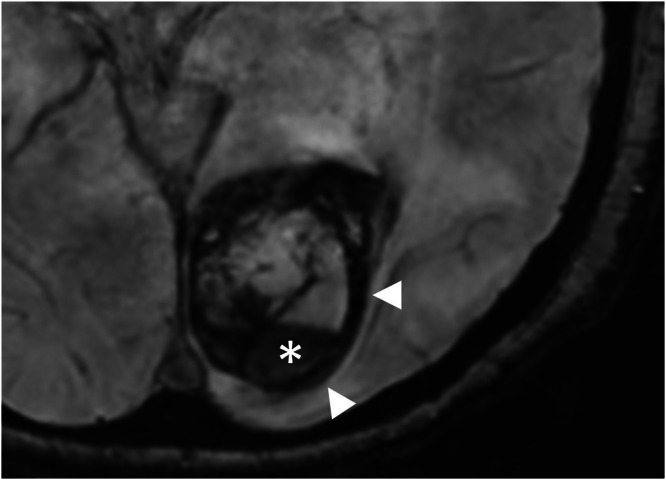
Susceptibility-weighted axial MRI image showing hypointense hemosiderin deposits surrounding the lesion (arrowheads) and a haemorrhagic fluid-fluid level (star).
